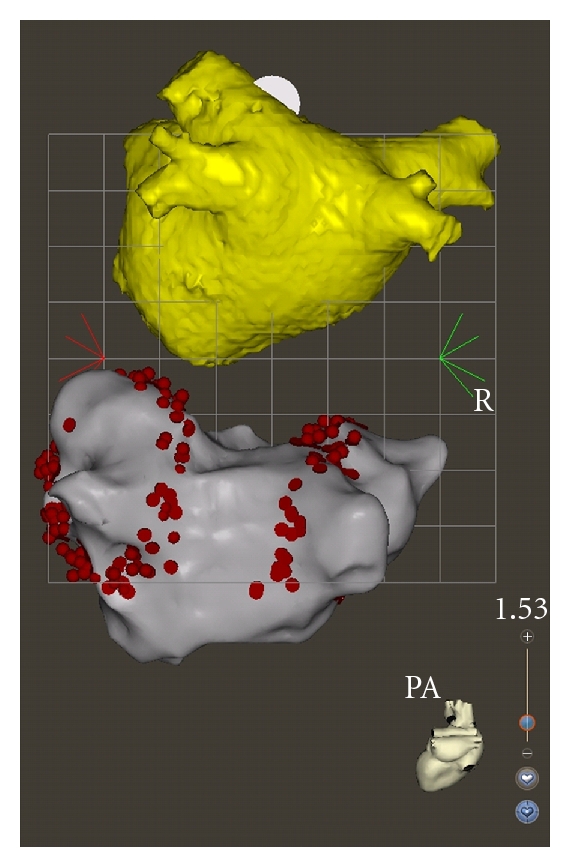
Three-dimensional reconstruction (yellow) of the computed tomography imaging and three-dimensional electro-anatomical map (grey) of the left atrium in posteroanterior projection with circumferential ablation (red points) around ipsilateral pulmonary veins using CARTO system (Biosense Webster Inc., Diamond Bar, Calif, USA).
